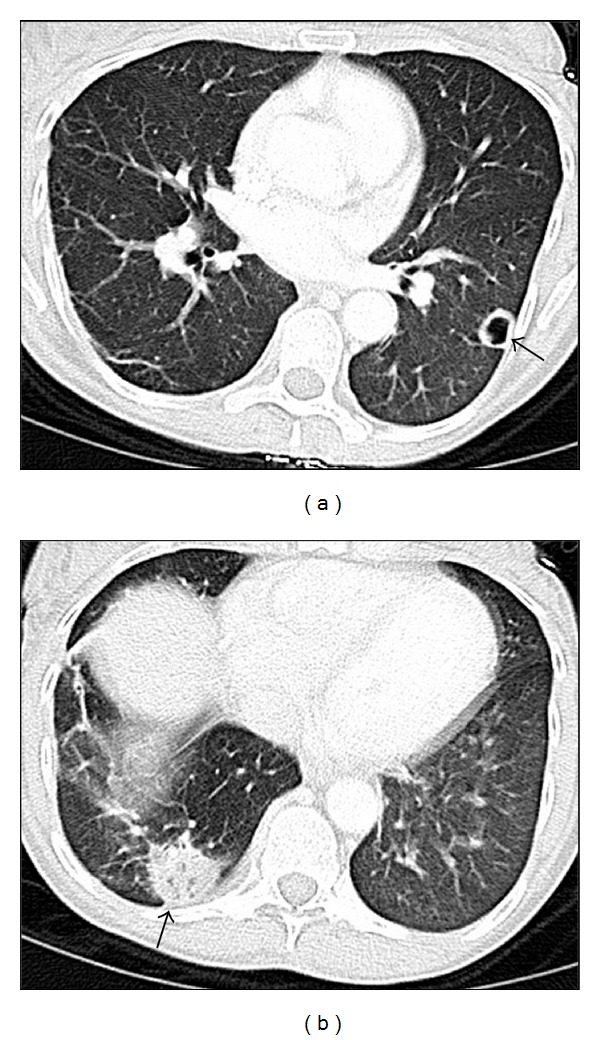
Chest CT shows a peripheral 1.7 cm cavitary nodule in the left lower lobe (a), and a peripheral 3 cm mass-like infiltrate in the right lower lobe (b).
